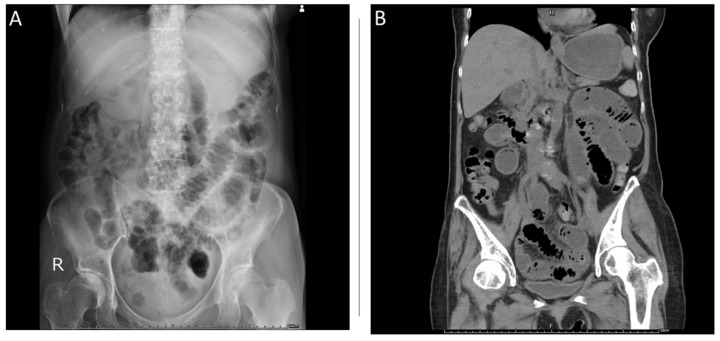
Abdominal plain view computed tomography (CT). (A) Abdominal plain view CT showing distention of small bowel loops with a coiled spring sign. (B) Abdominal CT showing generally dilated, air-fluid levels in the intestine, compatible with small intestinal obstruction.
